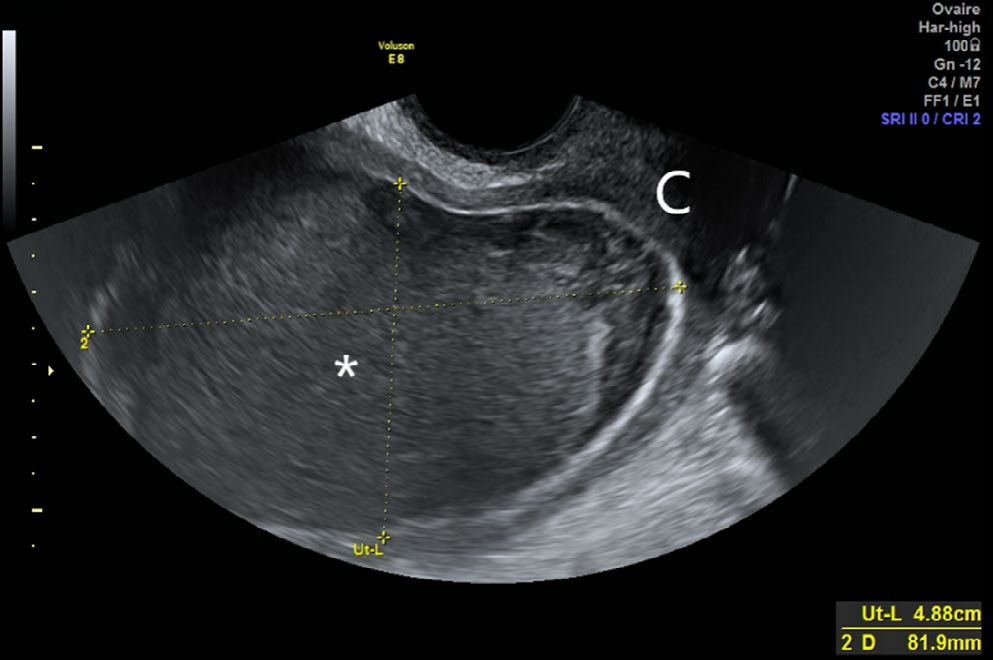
Pelvic ultrasonography sagittal view reveals an intrauterine cystic mass (81.9 mm length), suggesting hematometra or pyometra (*). C, uterine cervix
